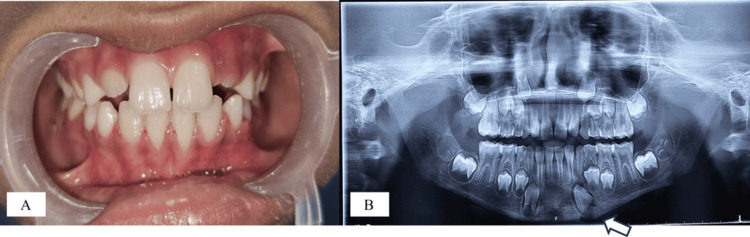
Two-month follow-up pictures: (A) clinical photograph after splint removal showing proper occlusion; (B) OPG after splint removal showing the initiation of calcification at the lost bone fragment site OPG: orthopantomogram
